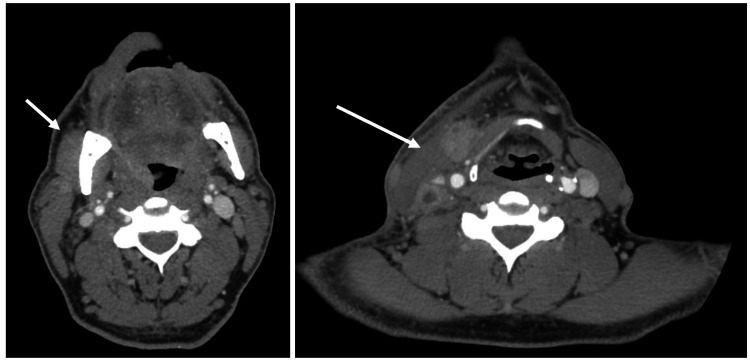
Cervical lymphadenopathy as shown by axial CT angiogram of the neck. Lymphadenopathy is shown by arrows in images.
